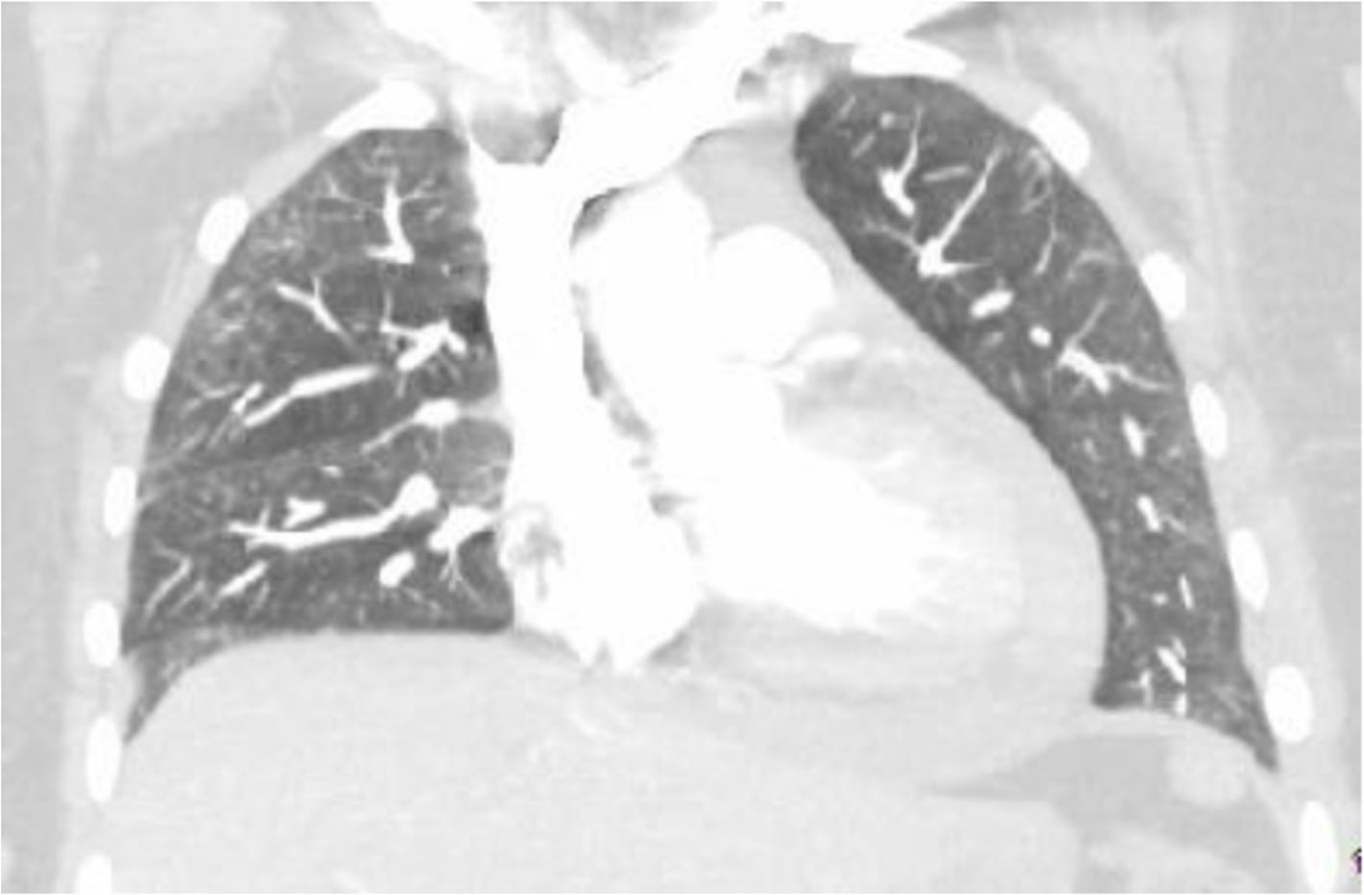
Repat CTA showing pericardial effusion
